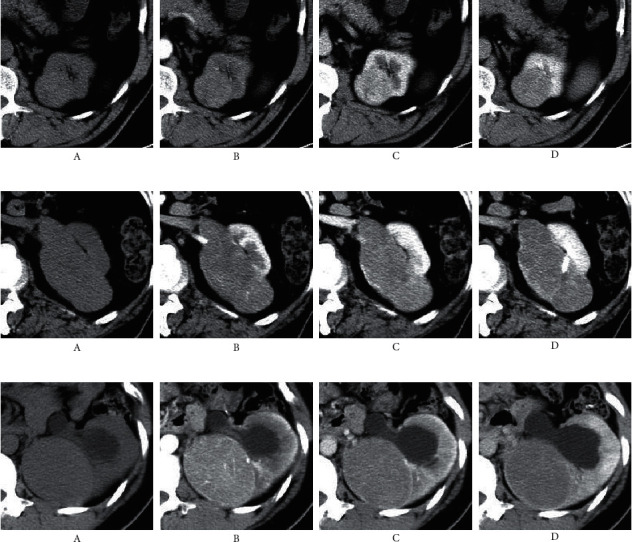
Enhanced computed tomography (CT) images of different subtypes of renal carcinoma in different phases. (a) Clear cell renal cell carcinoma; (b) papillary renal cell carcinoma; (c) chromophobe renal cell carcinoma. (A) Noncontrast phase; (B) corticomedullary phase; (C) nephrographic phase; (D) excretory phase.
